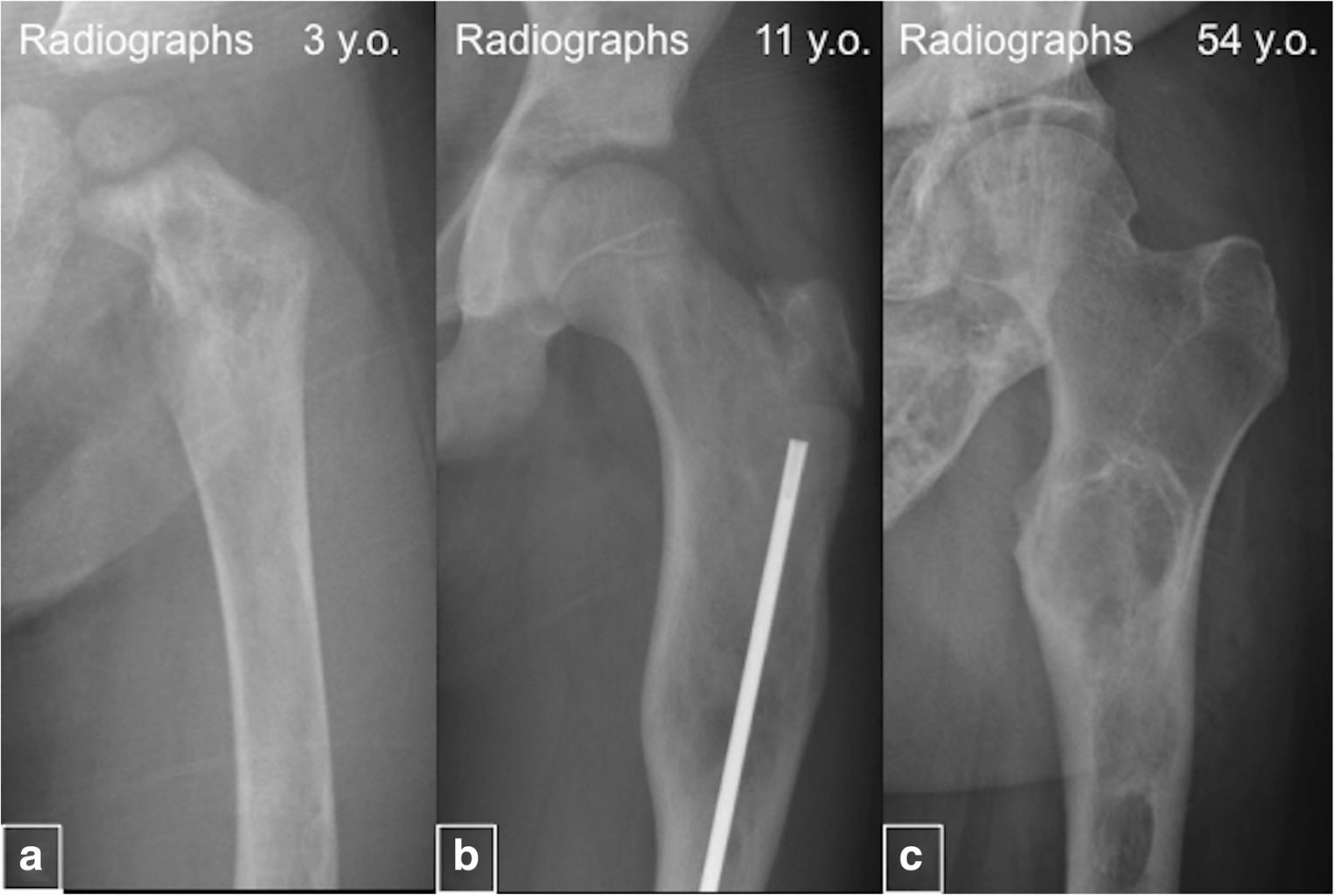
“Evolution” of the fibrous dysplasia (FD) lesions. a Radiograph of a 3-year-old demonstrates a typical heterogeneous-appearing FD lesion in the femur. b Radiograph from an 11-year-old demonstrates homogeneous and radiolucent FD lesion. c Image from a 54-year-old patient shows sclerotic FD lesions
